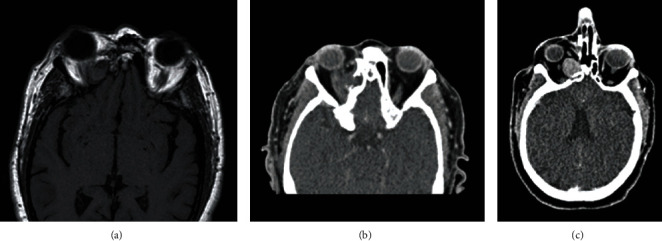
Case 2. (a) MRI in axial view showing the initial large, well-defined round mass over the superior and posterior orbits. Axial computed tomography (CT) imaging showing (b) the third recurrence which includes ethmoidal invasion 3 years later (c) and a fifth recurrence 5 years later.
